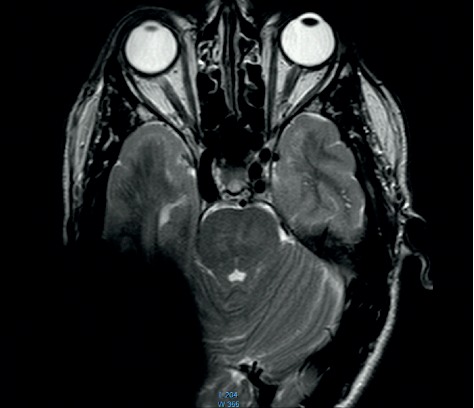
MRI pattern of the axial plain of a 1.5 T scanning Advanced Bionics 3D Implant.
